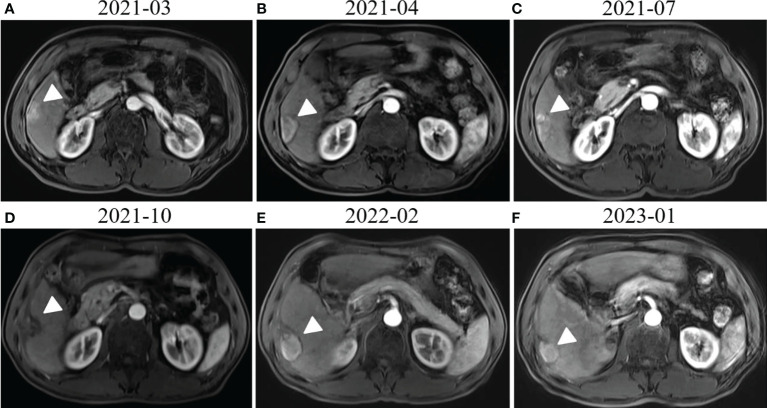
Radiological response evaluation of the liver during the clinical course. (A) Contrast-enhanced magnetic resonance imaging (MRI) revealed enhanced subcapsular hepatocellular carcinoma (HCC) lesions in the hepatic S6 (white arrowhead). (B) Contrast-enhanced MRI revealed that the lesion in hepatic S6 was not enhanced in the arterial phase (white arrowhead) 1 month after transcatheter arterial chemoembolization (TACE) and radiofrequency ablation (RFA). (C) An enhanced small nodule around the previous one (white arrowhead) was detected by MRI three months after TACE and RFA. (D) Contrast-enhanced MRI showed no active lesions in the liver (white arrowhead) 3 months after surgical resection of the liver metastases. (E) Complete response (CR) was confirmed by contrast-enhanced MRI, which showed no active lesions in the liver (white arrowhead) after RFA and four cycles of tislelizumab treatment. (F) MRI obtained 15 months after tislelizumab treatment initiation showed that there were still no active lesions (white arrowhead) in the liver or new lesion.
